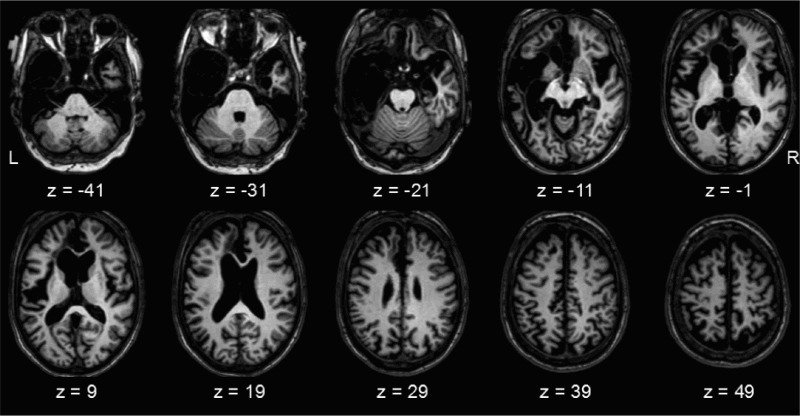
Anatomy of patient GA’s brain on a T1-weighted magnetic resonance image, normalized to MNI space using the clinical toolbox implemented in SPM8 (www.fil.ion.ucl.ac.uk/spm).
